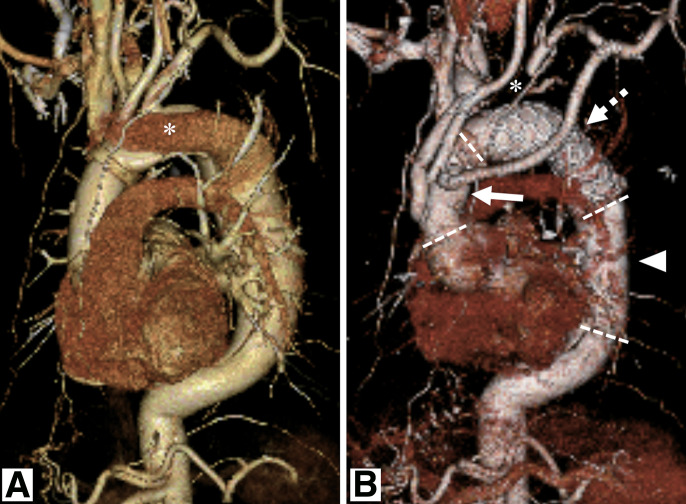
Contrast-enhanced CT images before and after aortic surgery. (A) Preoperative VR image prior to total arch replacement with frozen elephant trunk. An asterisk indicates contrast enhancement of the false lumen. (B) Postoperative VR image following the final surgery. Arrow: Branched prosthetic graft in the ascending aorta. Dashed arrow: Frozen elephant trunk graft. Arrowhead: Prosthetic graft in the descending aorta. Dashed lines: Anastomosis sites. Note that the left subclavian artery is ligated (*). CT: computed tomography; VR: volume-rendered
